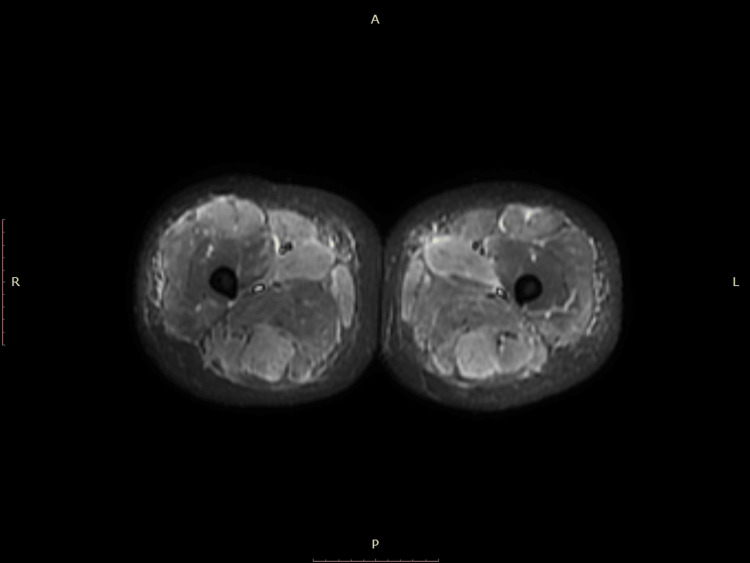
MRI axial STIR image of bilateral lower extremities showing muscle inflammation
